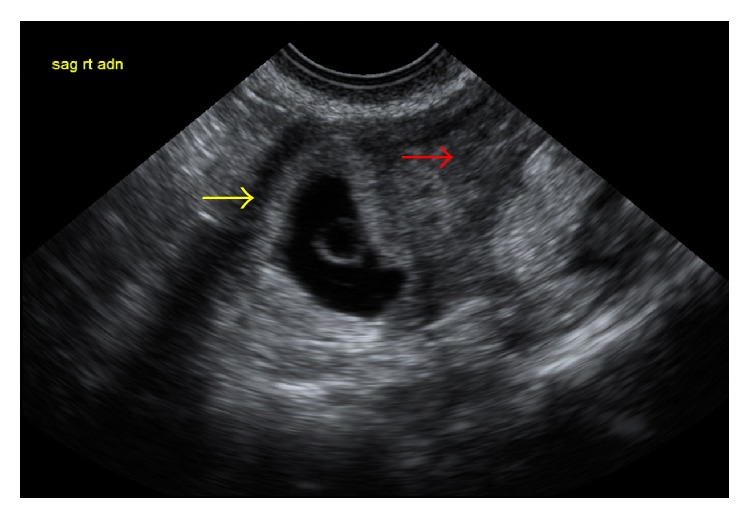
Transvaginal ultrasound. Red arrow: endometrial stripe of 2.4 cm and no visible intrauterine pregnancy. Yellow arrow: right adnexal mass/ectopic pregnancy.
